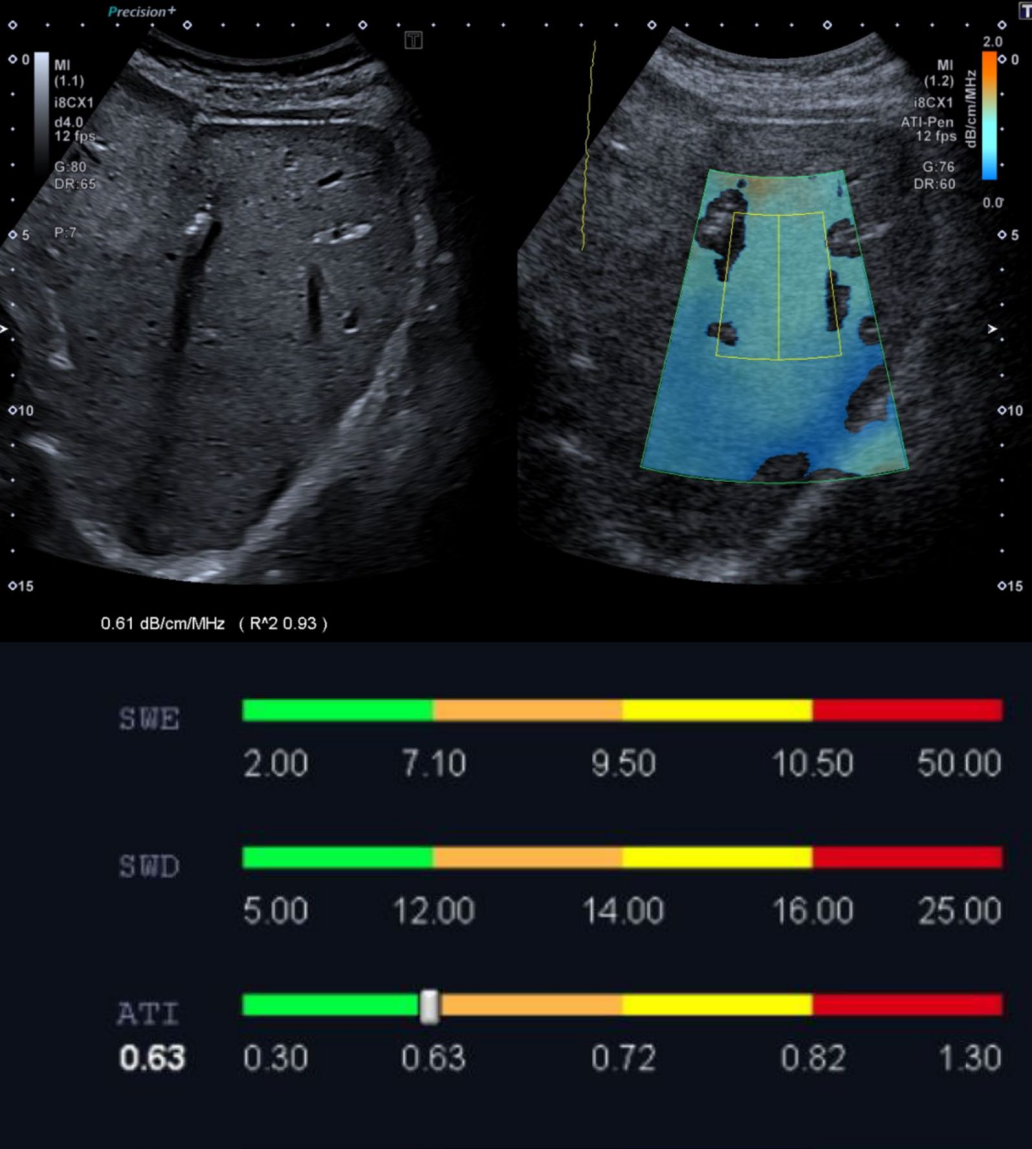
Attenuation imaging in the area of a B-scan sonographically inconspicuous liver region. The mean attenuation coefficient from five measurements is 0.63 dB/cm/MHZ
